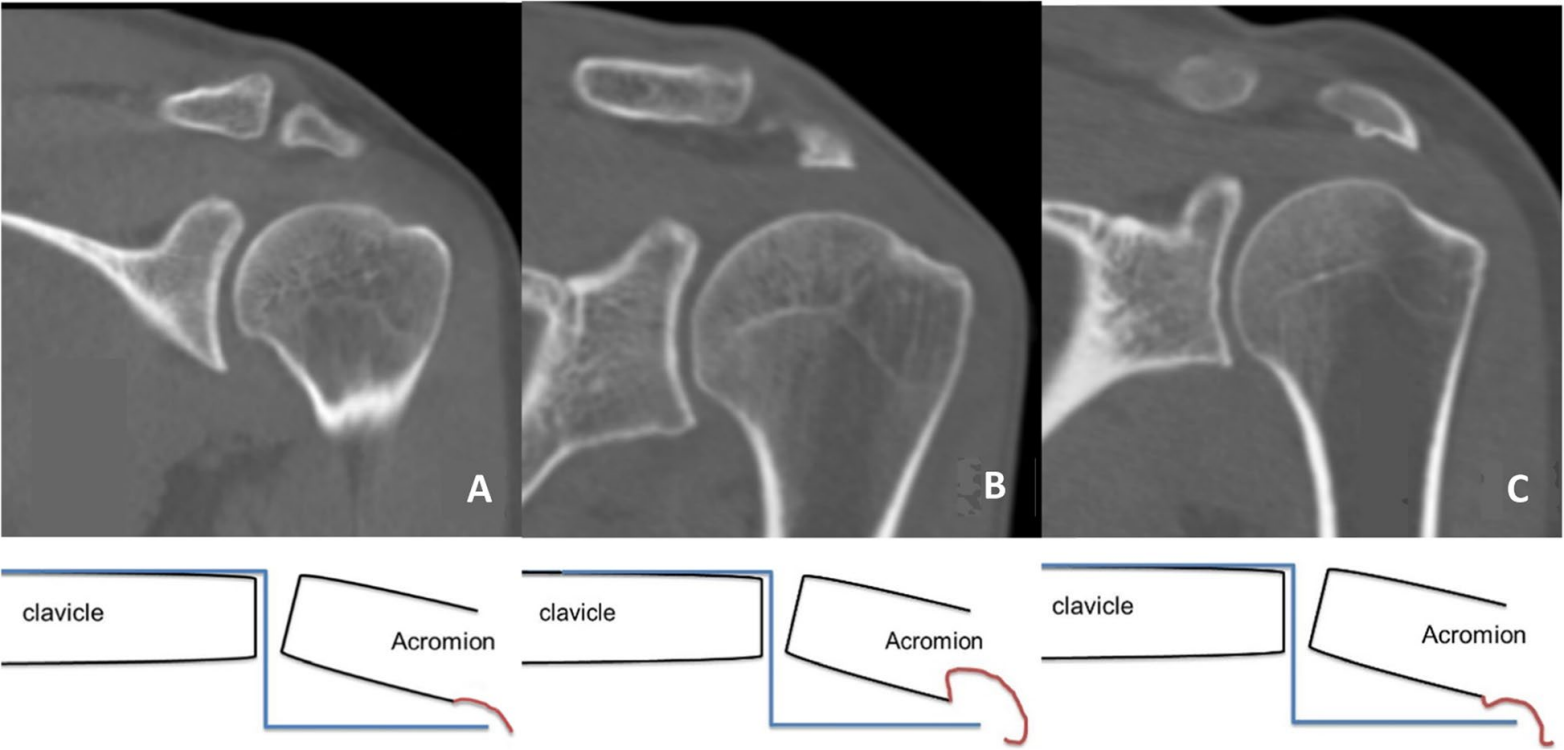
Computed tomography is used to decide acromial erosion type (A: Type I - simple groove, B: Type II - cave, C: Type III - marginal protrusion)
